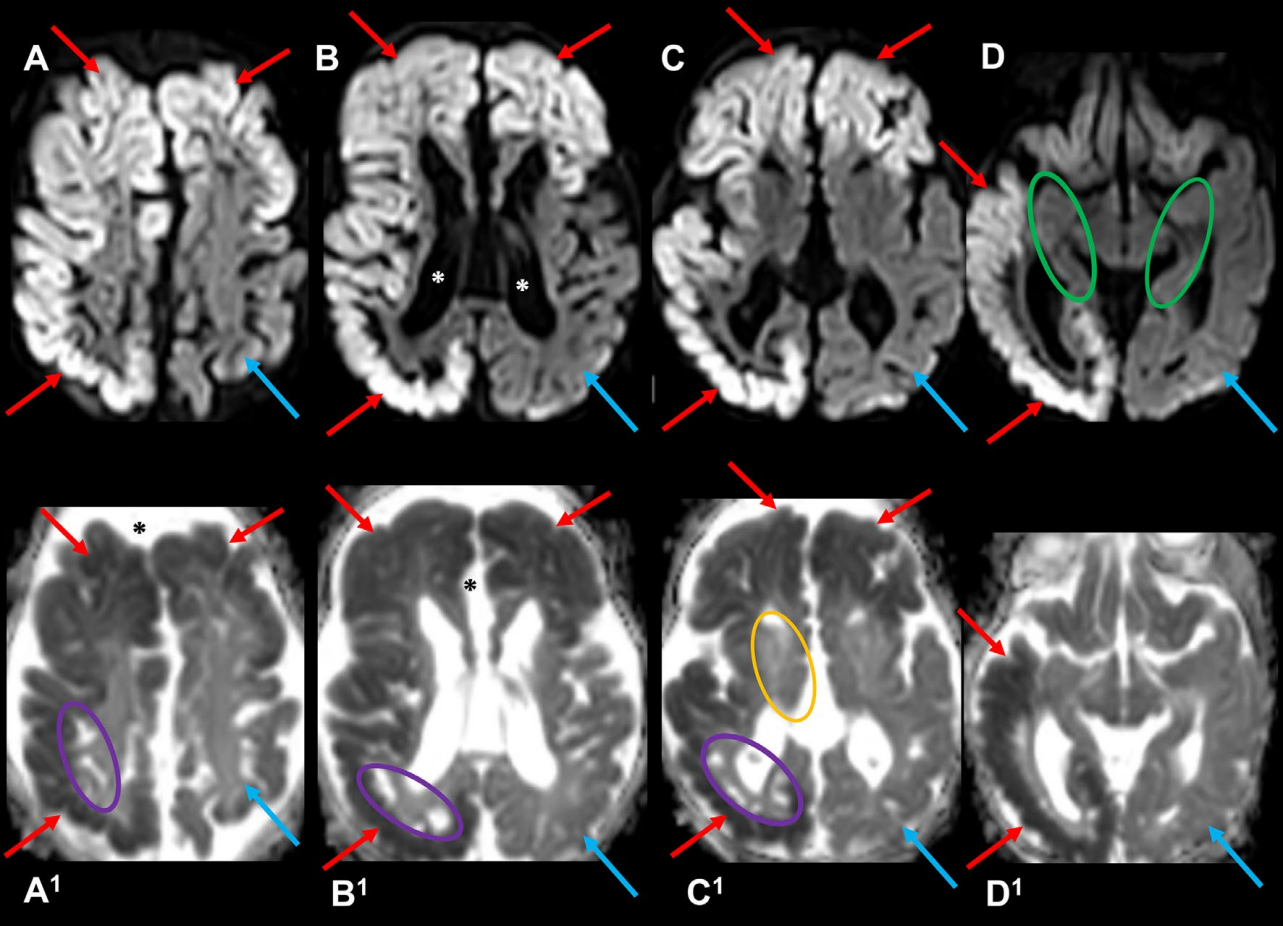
Asymmetric restricted diffusion superimposed on established brain atrophy and cystic encephalomalacia in Patient H. Upper row (A–D): axial DWI at age 42 days. Bottom row (A^1^–D^1^): Corresponding axial ADC map. High DWI/low ADC signal denotes cortical and subcortical WM cytotoxic edema in the right cerebral hemisphere and left frontal lobe (red arrows). No restricted diffusion is seen in the left‐sided parietal, temporal, and occipital lobes (blue arrows). Mesial temporal lobes are preserved (green ovals). Dilated lateral ventricles (white asterisks) and enlarged pericerebral CSF subarachnoid spaces (black asterisks) indicate brain atrophy. Note basal ganglia and thalamic atrophy (orange oval). Established cystic transformation is demonstrated in the parietal, temporal, and occipital subcortical WM (violet ovals).
